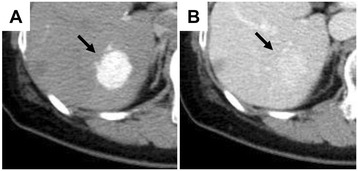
Computed tomography (CT) imaging of the abdomen. A. There is a tumor at the segment 6 of the liver, which is well enhanced by contrast medium at the arterial phase (*arrow*). B. The tumor remains to be enhanced by contrast medium in portal phase (*arrow*).
